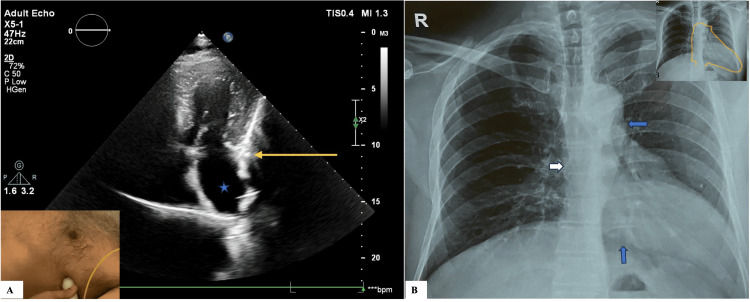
Echocardiography and X-ray radiography imaging A: A modified apical four-chamber view was obtained in the supine position with the transducer positioned near the posterior axillary line (box on bottom left corner). Elongated atria (star) with a widened ventricle demonstrating a classic ‘tear drop’ appearance were observed. The yellow arrow points to the abnormal sharp angulation between the atria and the ventricle. B: Chest radiograph demonstrating leftward cardiac displacement with obscuration of the right heart border (white arrow) and straightening of the left cardiac margin (‘Snoopy sign’ seen in the image on the top right corner). Also, a lucent area is seen between the aorta and pulmonary artery and between the left hemidiaphragm and the base of the heart (blue arrow).
